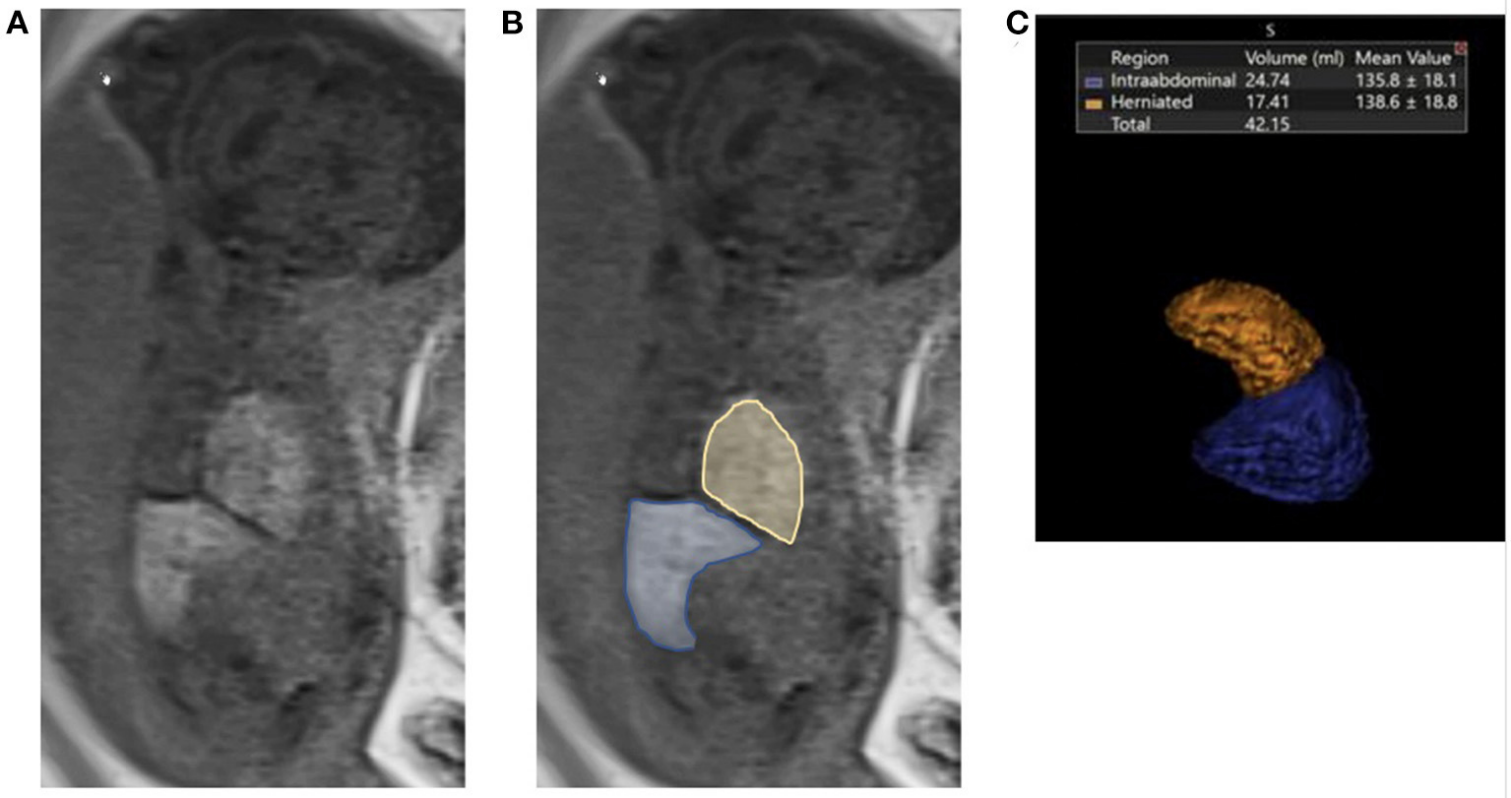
Fetal liver volumetry using T1 MRI images. (A) Liver down (blue) and liver up (yellow) volumes were determined on each MRI section by using a free-form region of interest on a Vitrea workstation (Vital Images Inc, Minnetonka, MN). (B) Delineation of the liver up and liver down on the ipsilateral site of defect was determined by mirroring the contour of the contralateral diaphragm. Liver volumes were then calculated in the T1-weighted coronal plane by using sequences that allowed complete imaging of the liver and without artifacts from motion and the summation. (C) Three dimensional representation of the liver volumes from the same patient.
